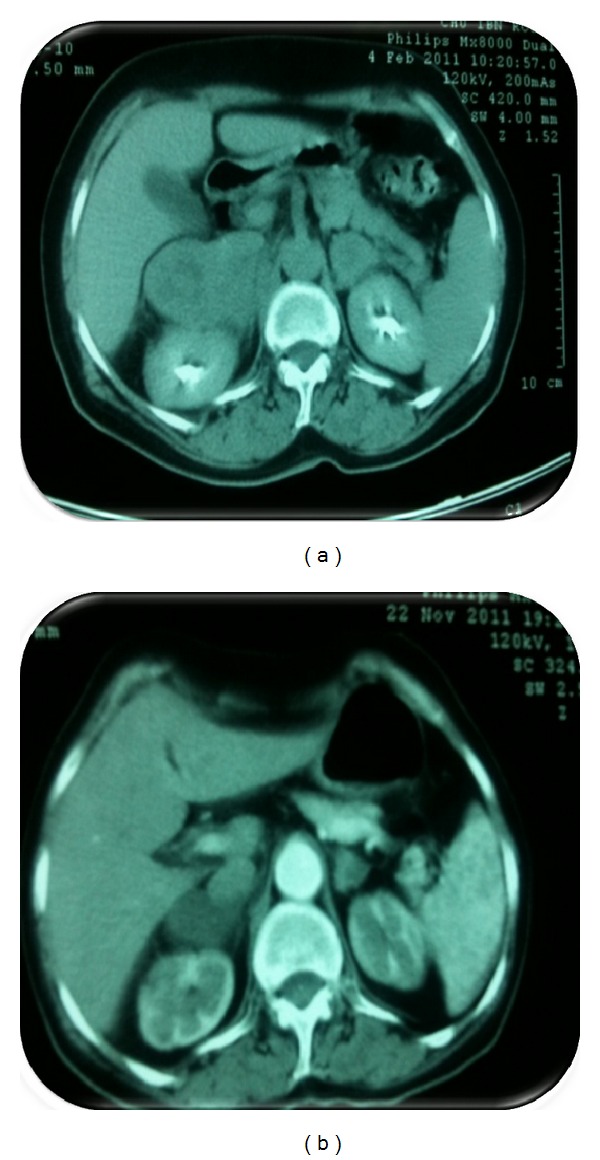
(a) Computed tomography of the abdomen shows a bilateral adrenal mass. (b) CT evaluation showed response of the adrenal metastases after chemotherapy.
